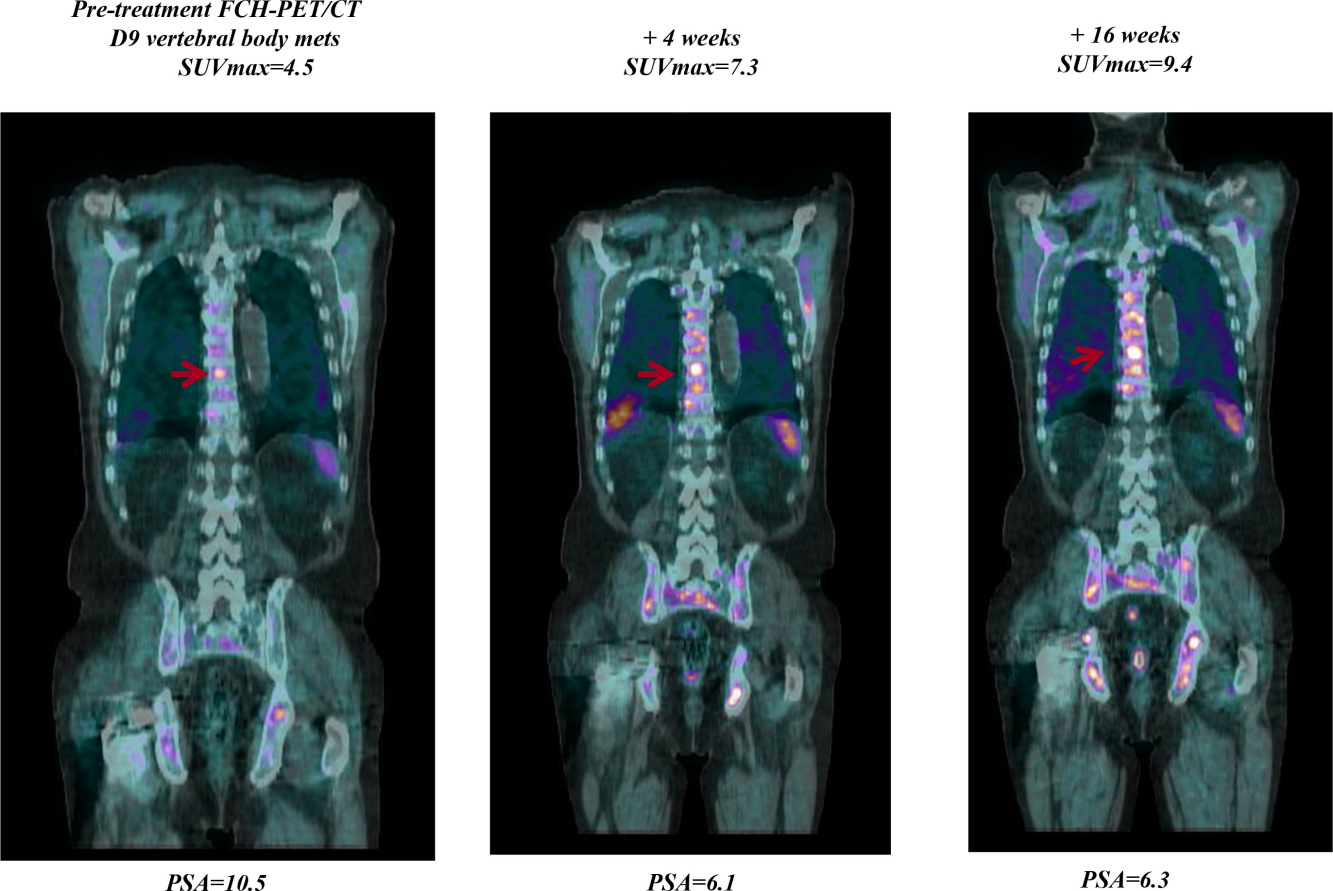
Early FCH-PET-CT progression on abiraterone treatment Baseline FCH-PET/CT shows multiple areas of increased radiotracer uptake indicative of extensive bone metastases. Treatment with abiraterone resulted in bone progression of FCH-PET/CT scans at week 4 confirmed at week 16.
